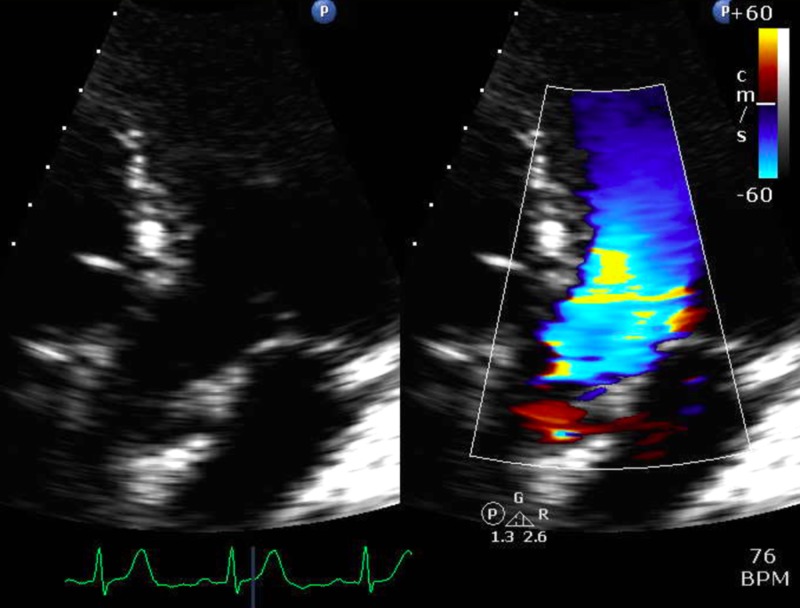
Next-day transthoracic echocardiographic image without left ventricular outflow tract obstruction
